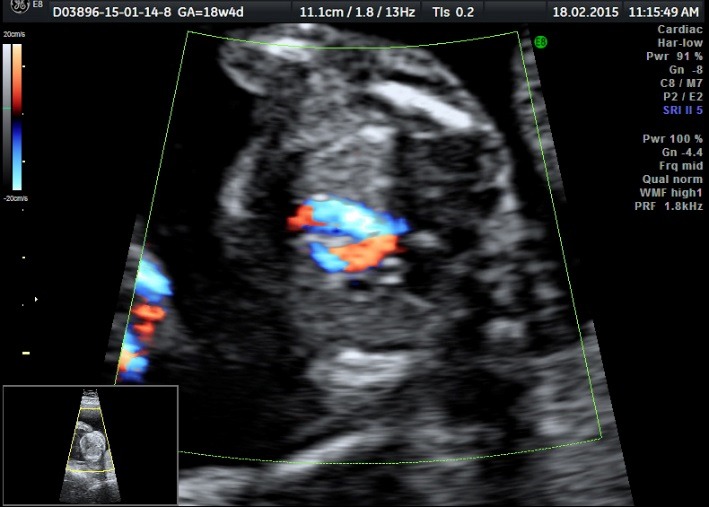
Normal fetus (pumping twin) with a normal heart – Doppler examination of the 3 vassel viewaryotype
